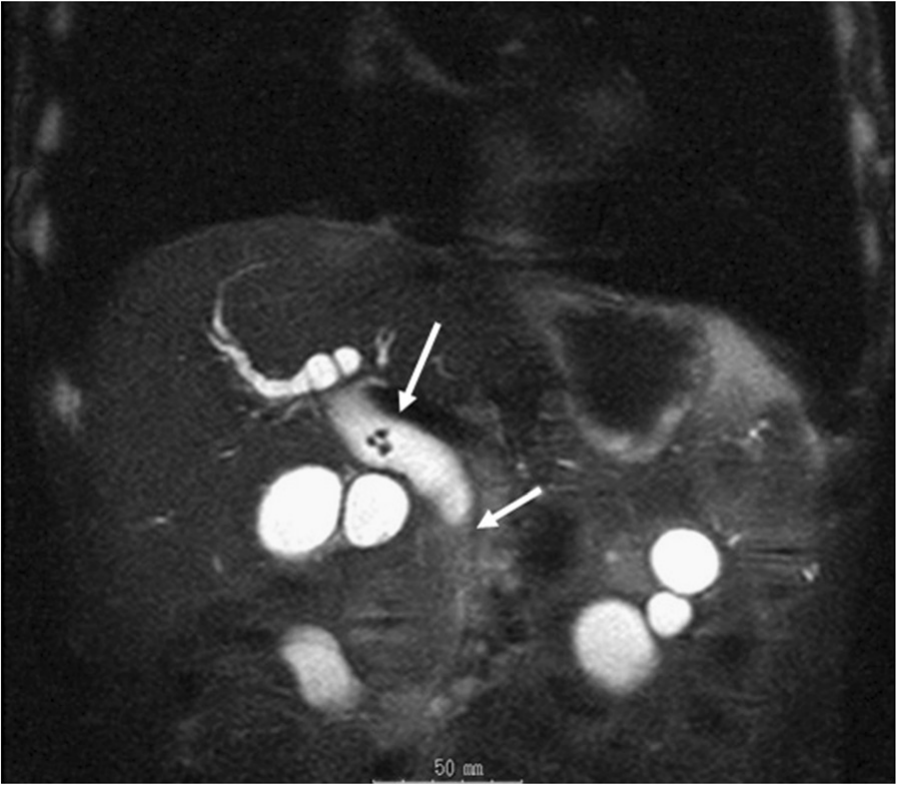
Abdominal magnetic resonance image (T2) taken at the time of admission. The arrows indicate bile duct stones.
